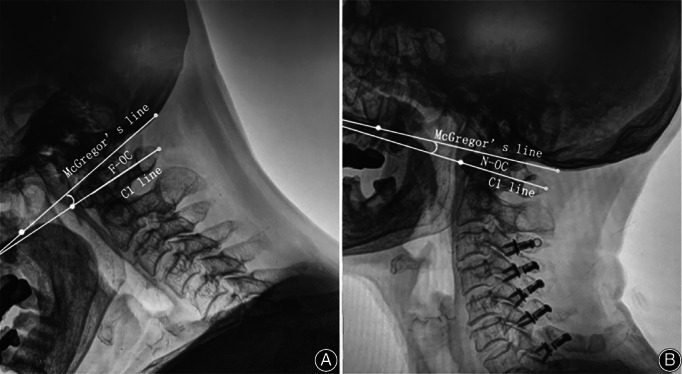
Representation of the radiographic measurement of O‐C_1_ angle in flexion position (A) and neutral (B) from the lateral view. The O‐C_1_ angle was measured between the McGregor's line and the line passing through the center of the C_1_ anterior arch and the center of the C_1_ posterior arch.
